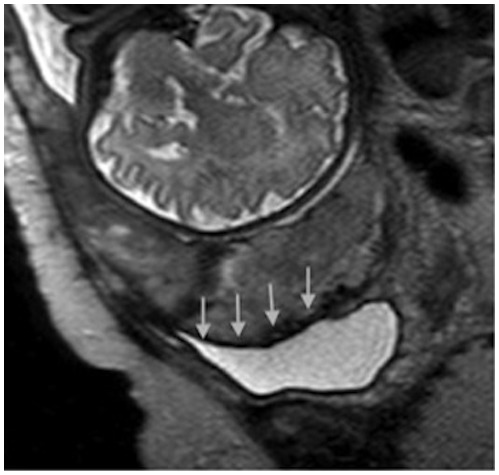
Uterine bulging into the bladder on MRI.
